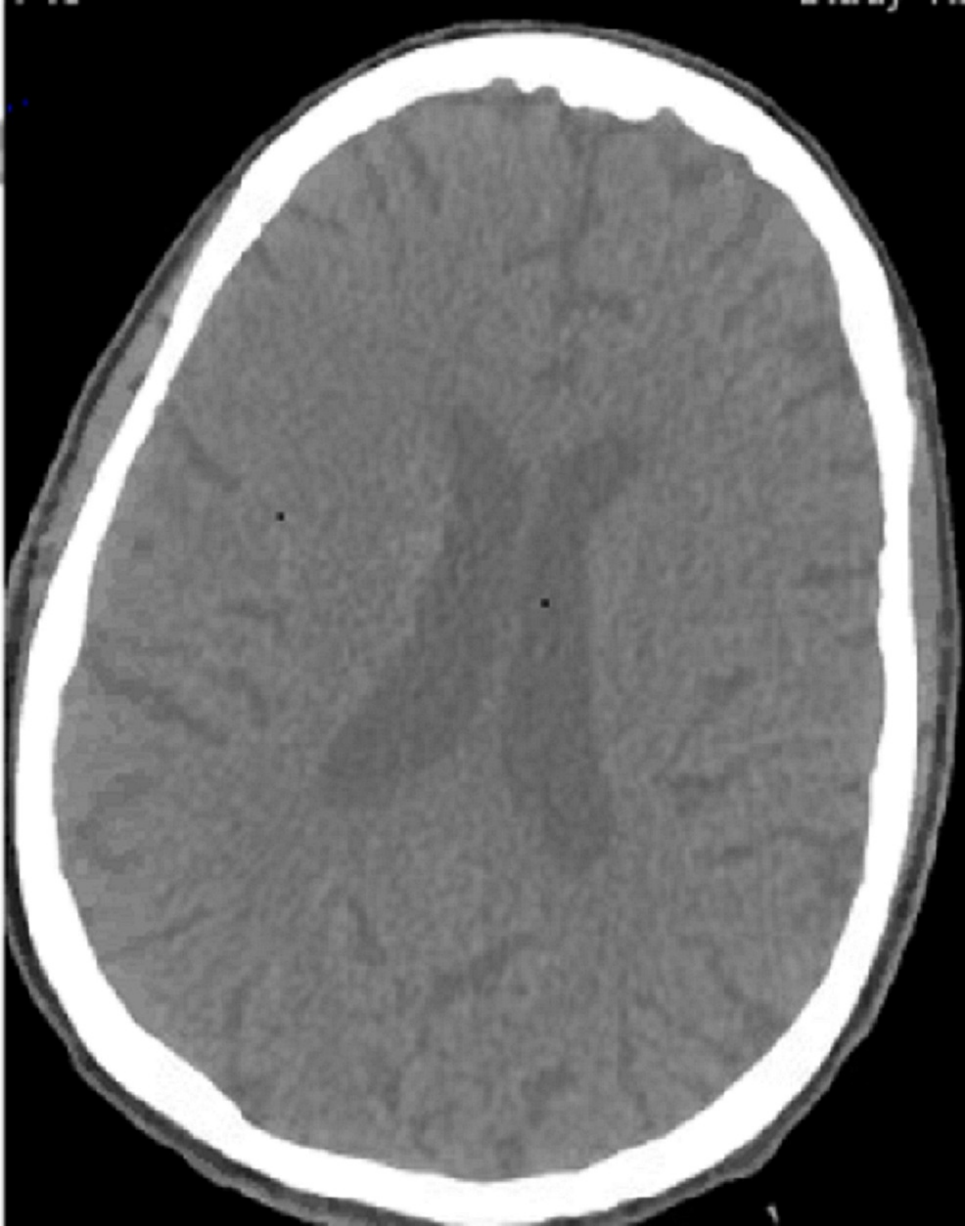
CT scan of the head showing no acute pathology
